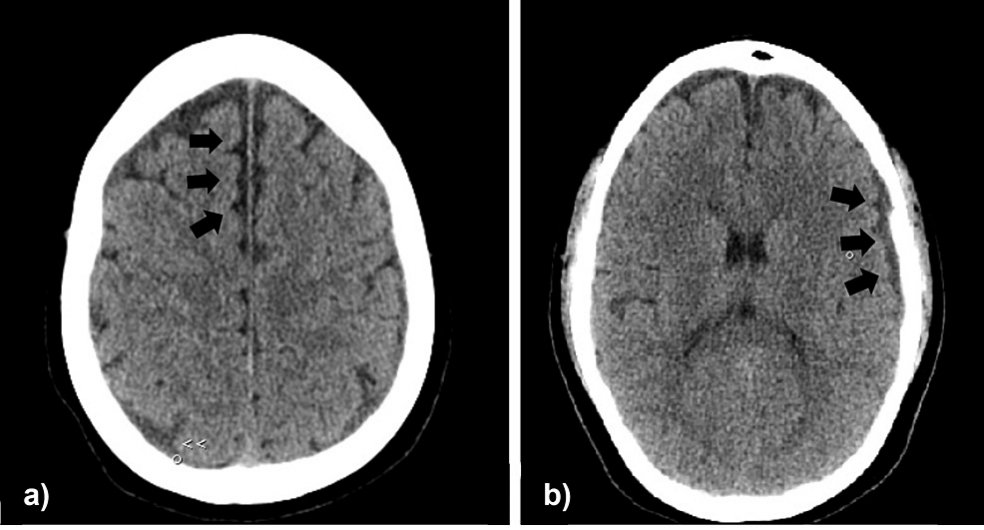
Cranial CT showing (a) parasagittal parietal and (b) frontoparietal hygromas (black arrows).
